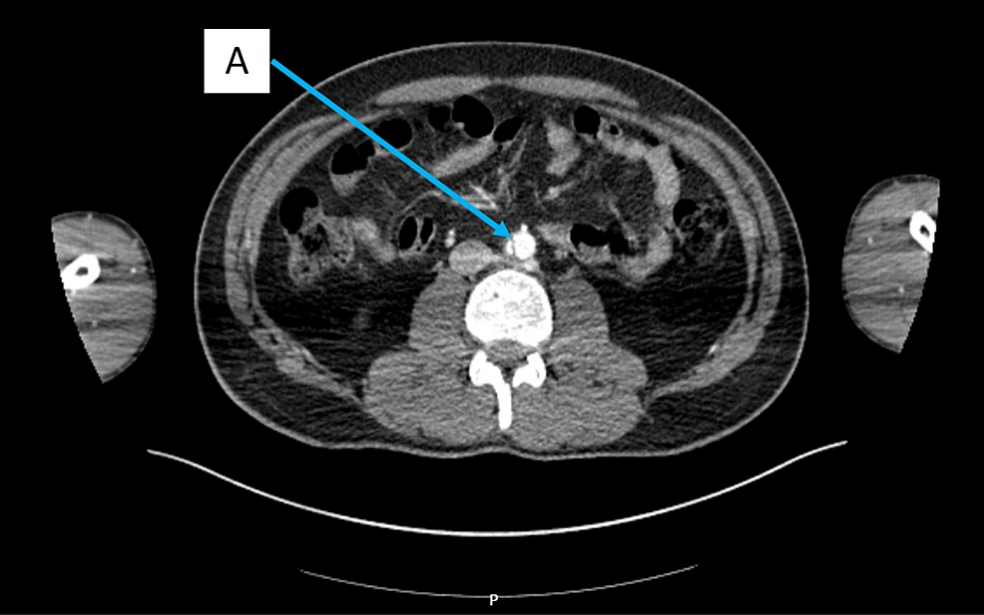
Axial section computed tomography (CT) imaging with contrast showing an intimal tear and aortic dissection (A) in the infrarenal abdominal aorta.
